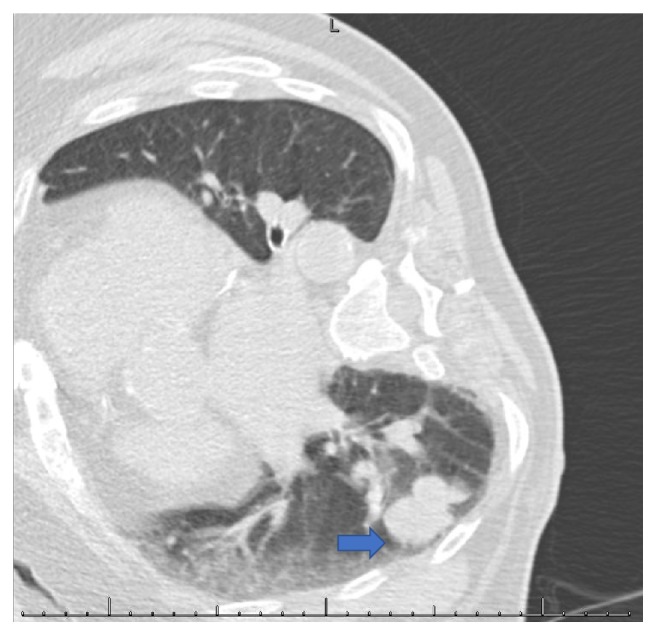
Chest CT. Arrow: 3.2x2x4.3 cm mass in the superior segment of the right lower lobe.
